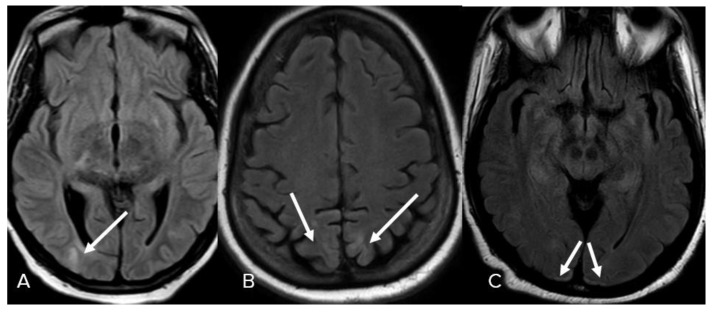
Cortical involvement. (A) Axial FLAIR images demonstrate isolated cortical-based FLAIR hyperintensity in the right occipital lobe. (B) Bilateral parietal FLAIR hyperintensities. (C) Bilateral occipital FLAIR hyperintensities. Image has been windowed manually to improve the visibility of signal abnormality.
